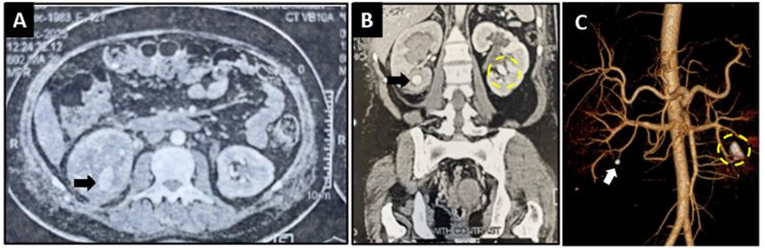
(A) Axial post-contrast abdominal CT scan demonstrates right side renal artery pseudoaneurysm (black arrows). (B) Coronal post-contrast image also shows the pseudoaneurysm (black arrows) and a non-obstructive stone is noted at lower pole of left kidney (dotted yellow circle). (C) In 3D reconstructed image, angio-embolization was done and embolization coil is shown (white arrow) as well as the mentioned left renal stone (dotted yellow circle).
